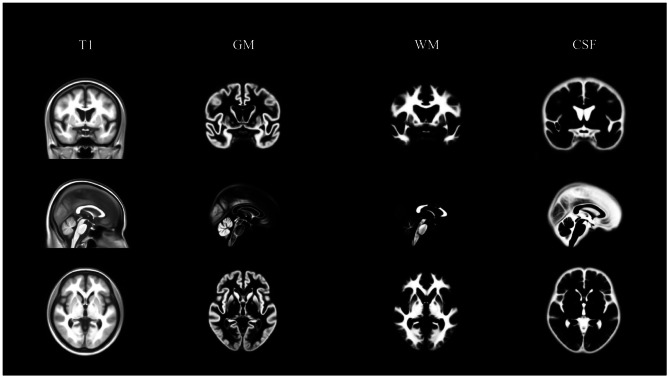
KNE200 brain MRI template
